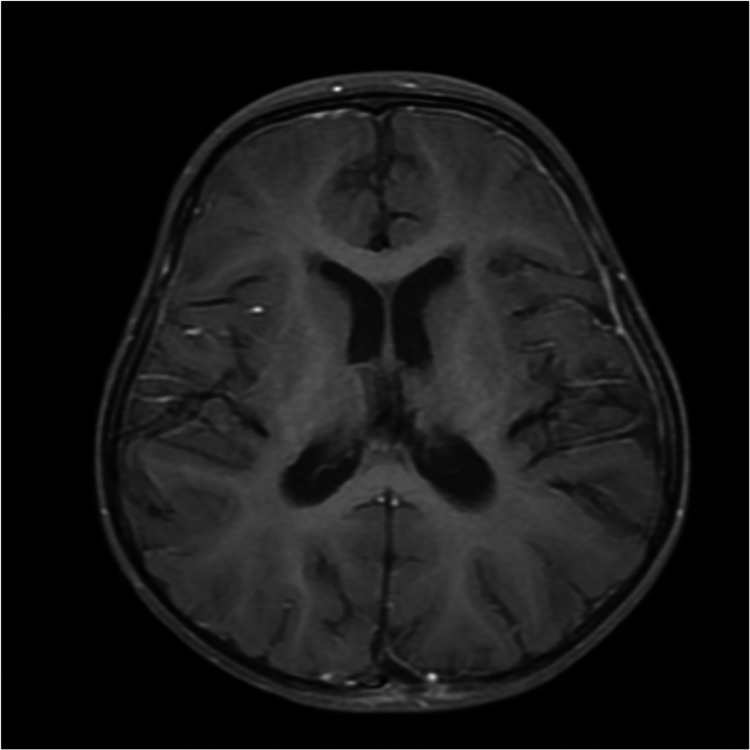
Follow-up brain MRI axial view showing nearly complete resolution of the brain abscesses six weeks after treatment.
